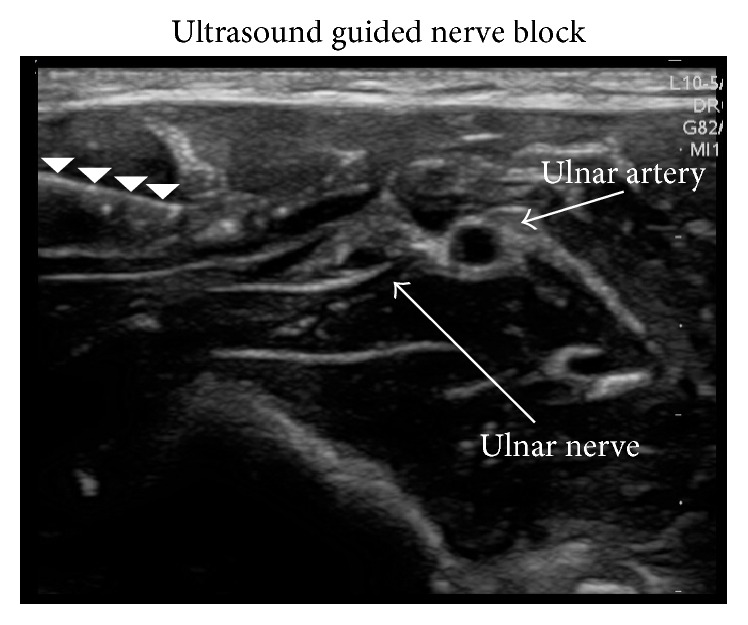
Ultrasound image demonstrating needle and anesthetic solution around the ulnar nerve.
